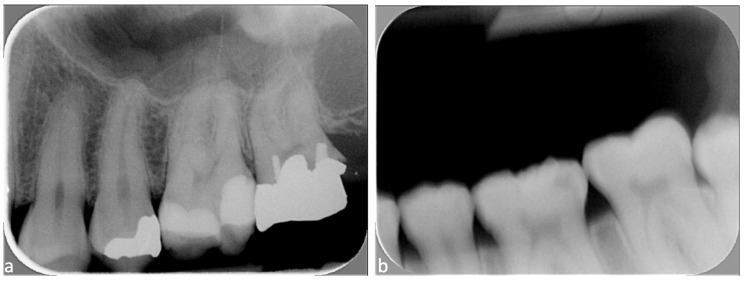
Periapical radiograph scores presented from left to right: (a) score 11/13: acceptable X-ray; and (b) score 3/13: unacceptable X-ray.
